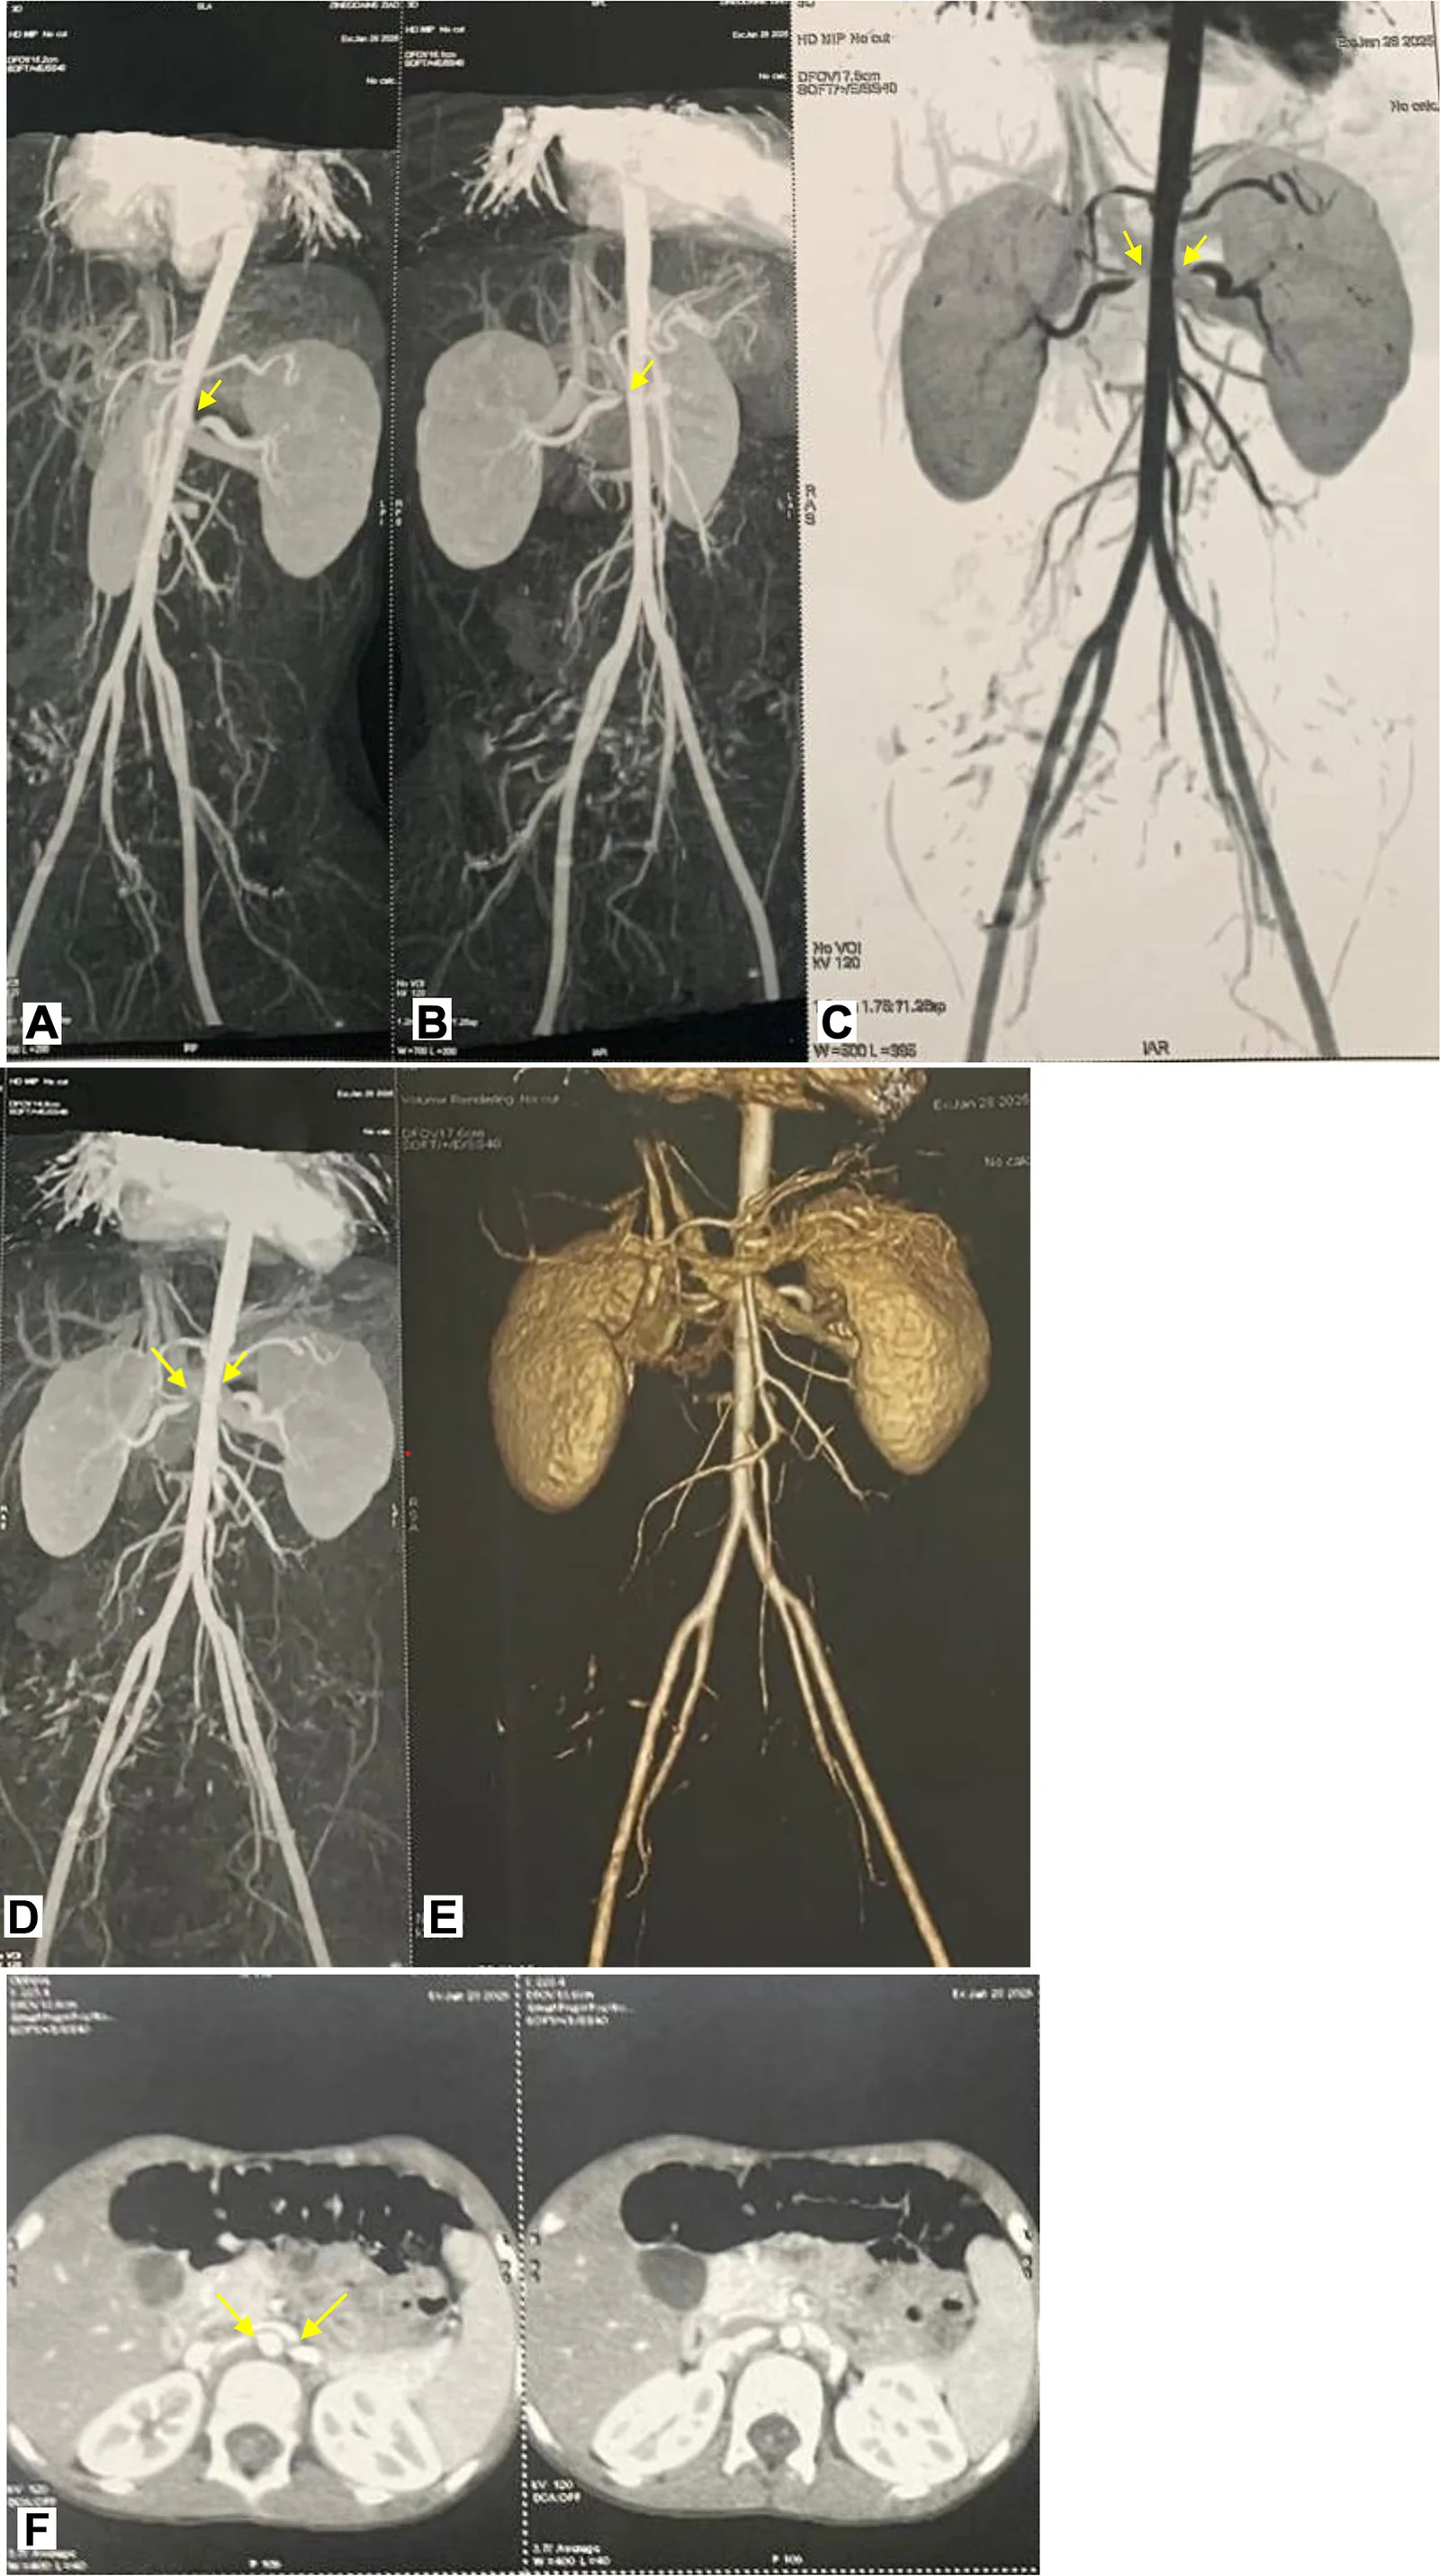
CT angiography confirmed severe, symmetrical bilateral renal artery stenosis involving the ostial and proximal segments, with an estimated luminal narrowing greater than 90%. (A–D) Coronal maximum-intensity projection (MIP) reconstructions obtained following contrast-enhanced CT angiography. Yellow arrows indicate severe bilateral ostial and postostial renal artery stenoses. Distal renal arterial segments remain patent with preserved opacification of the segmental and interlobar branches. (E) Three-dimensional volume-rendered (VRT) reconstruction demonstrating bilateral narrowing at the origins of the renal arteries arising from an otherwise normal abdominal aorta. (F) Axial contrast-enhanced CT angiographic image showing marked narrowing of both renal artery ostia (yellow arrows). These findings correlate with the Doppler ultrasound examination, which demonstrated absent measurable flow at the ostial and proximal renal artery segments, supporting the diagnosis of severe bilateral proximal renal artery stenosis.
